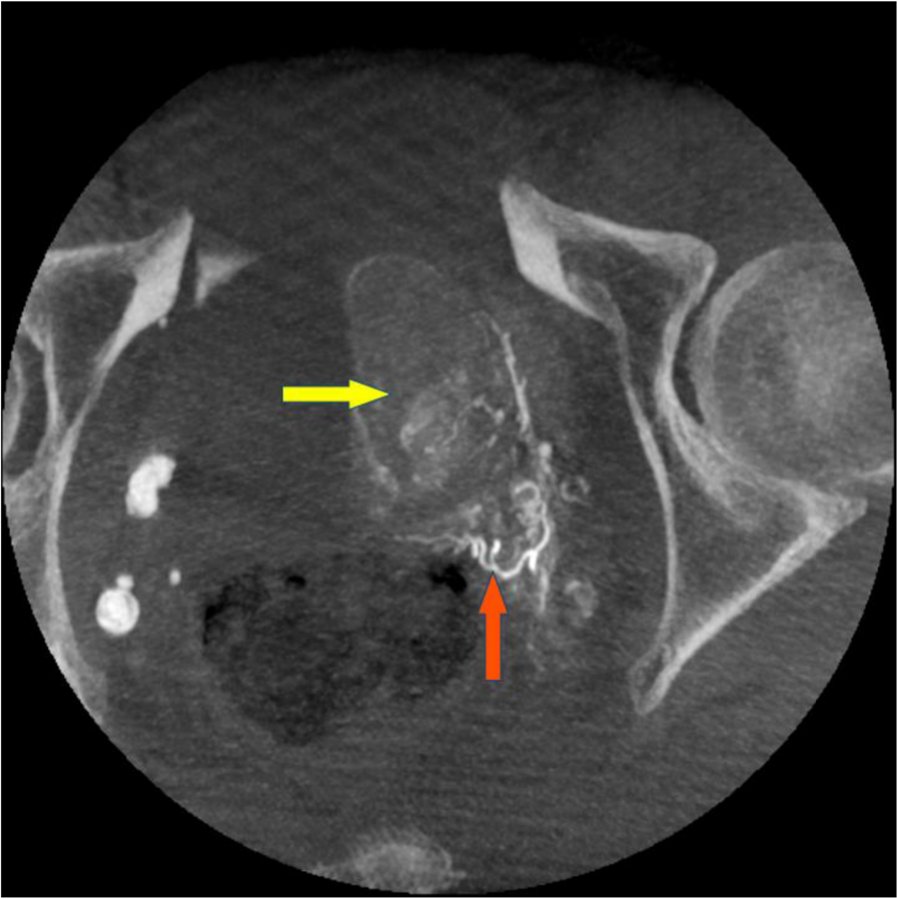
Distal CBCT. Ten millimeters MIP axial view from a super-selective CBCT showing prostatic blush (yellow arrow) as well as rectal branch (orange arrow) originating from a prostatic artery, observed after vasodilator injection. The catheter had to be repositioned to avoid off-target embolization
